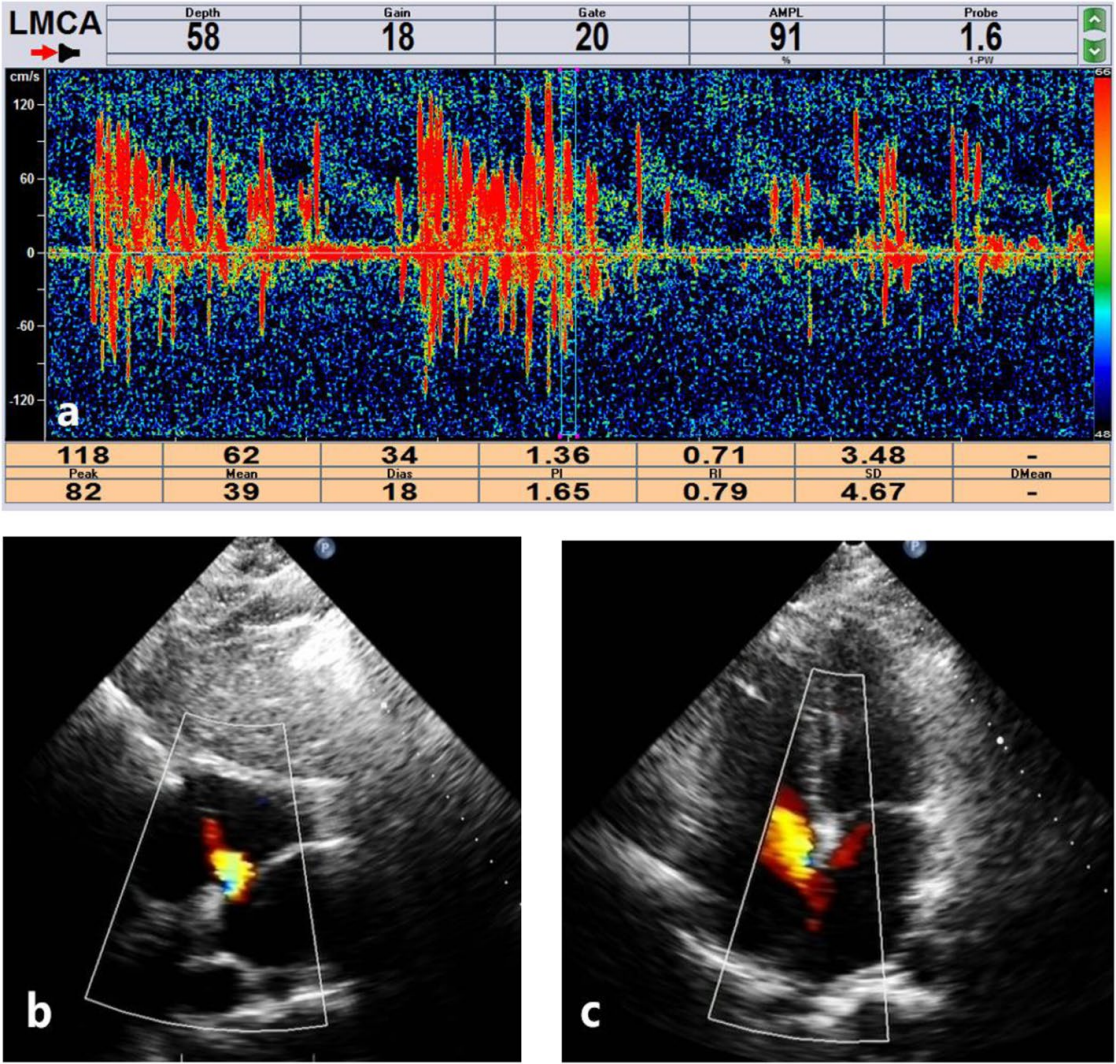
a Transcranial Doppler (TCD) result showing high-intensity transient signals, several of which masked background waveforms. b and c Transthoracic echocardiography (TTE) images of patent foramen ovale (PFO)
